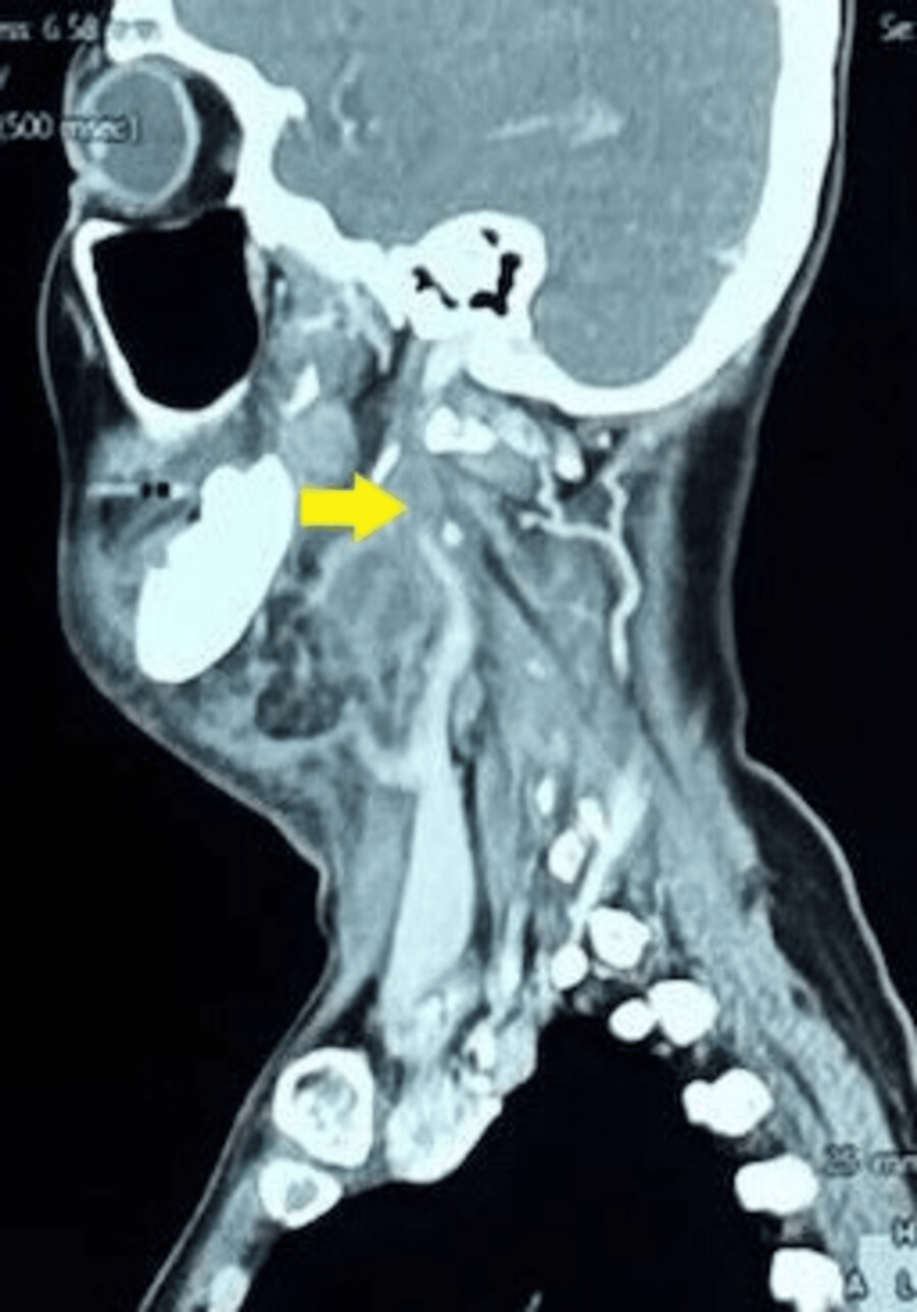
CT neck shows a rim-enhancing collection in the right parapharyngeal and submandibular spaces with surrounding inflammation, causing compression and poor visualization of the right IJV, raising suspicion for thrombosis (indicated by arrow) IJV: Internal jugular vein
